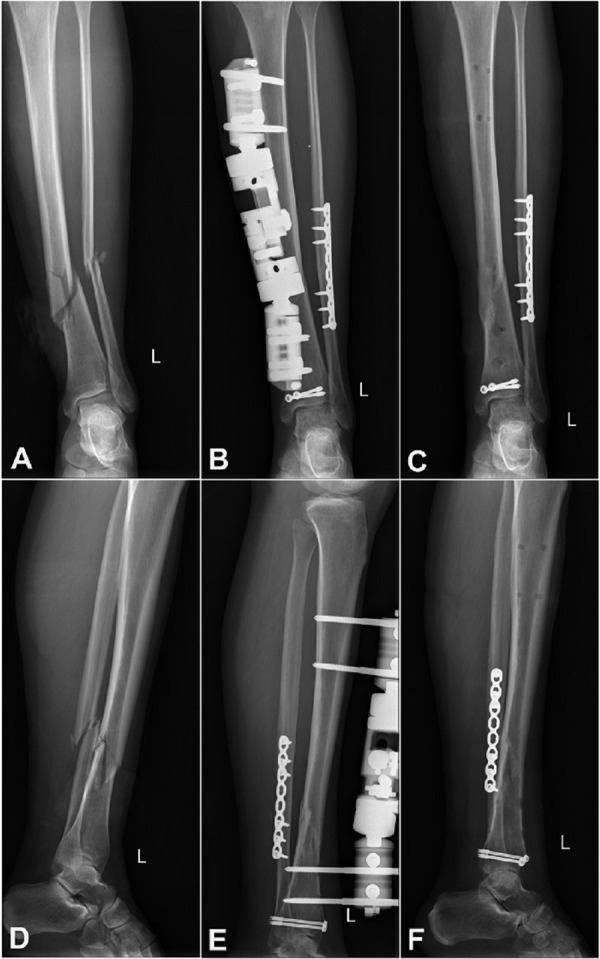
A, D: Comminuted fracture of tibia and fibula. B, E: X-ray showed some osteotylus across fracture line with good alignment 1 month after TCM treatment. C, F: Union of fracture without replacement after external fixation was removed.
